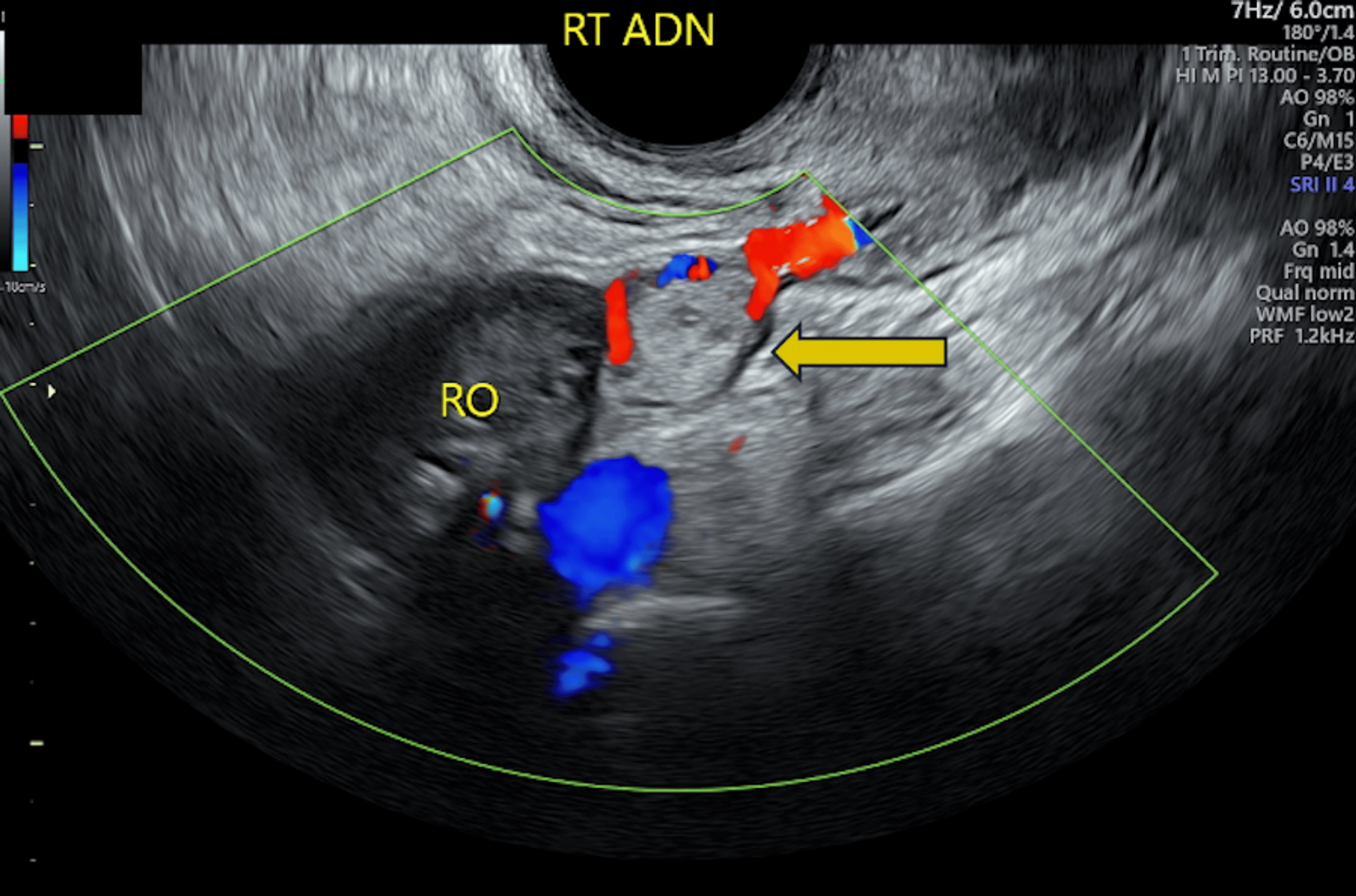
Transvaginal Doppler duplex ultrasound of the right adnexa. Complex cystic area with circumferential Doppler flow within the right adnexa measuring 1.32 x 1.20 cm without evidence of free fluid (yellow arrow). RO: right ovary; RT ADN: right adnexa.
